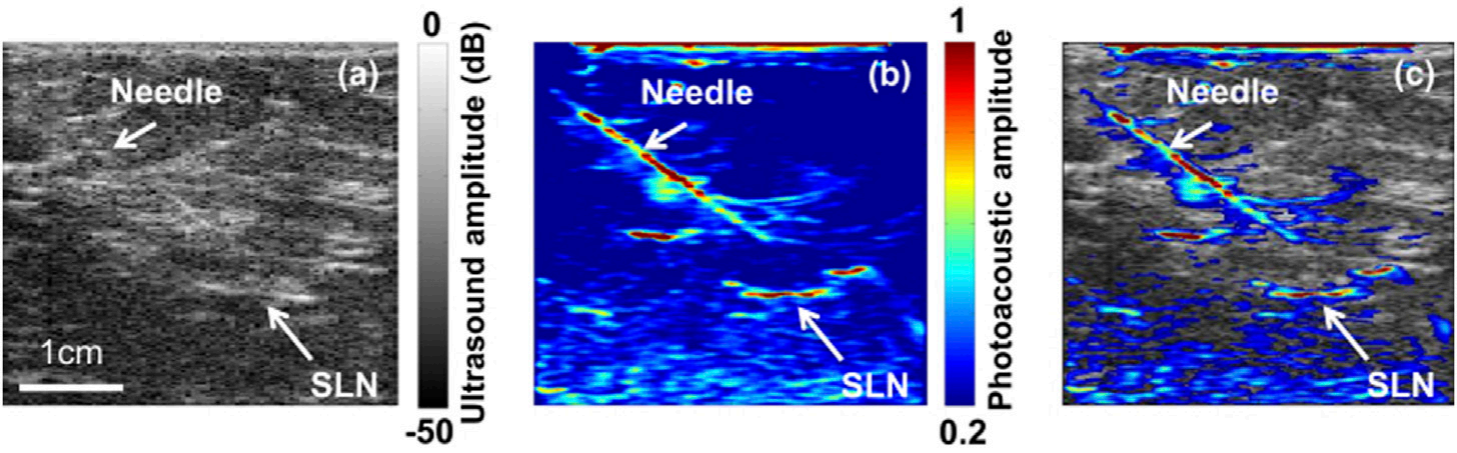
Images of the SLN and the needle acquired using PAT/US *in vivo*. (A) US image showing the lymph node and needle *in vivo*. (B) PAT image of the SLN and needle *in vivo*. (C) Coregistered PAT/US image of the SLN (long white arrow) and needle (short white arrow). SLN: sentinel lymph node; PAT: photoacoustic tomography; US: ultrasound. Reprinted with permission from [Garcia-Uribe A., et al. (2015). Dual-Modality Photoacoustic and Ultrasound Imaging System for Noninvasive Sentinel Lymph Node Detection in Patients with Breast Cancer. Sci Rep.5, 15748. doi: 10.1038/srep15748].
